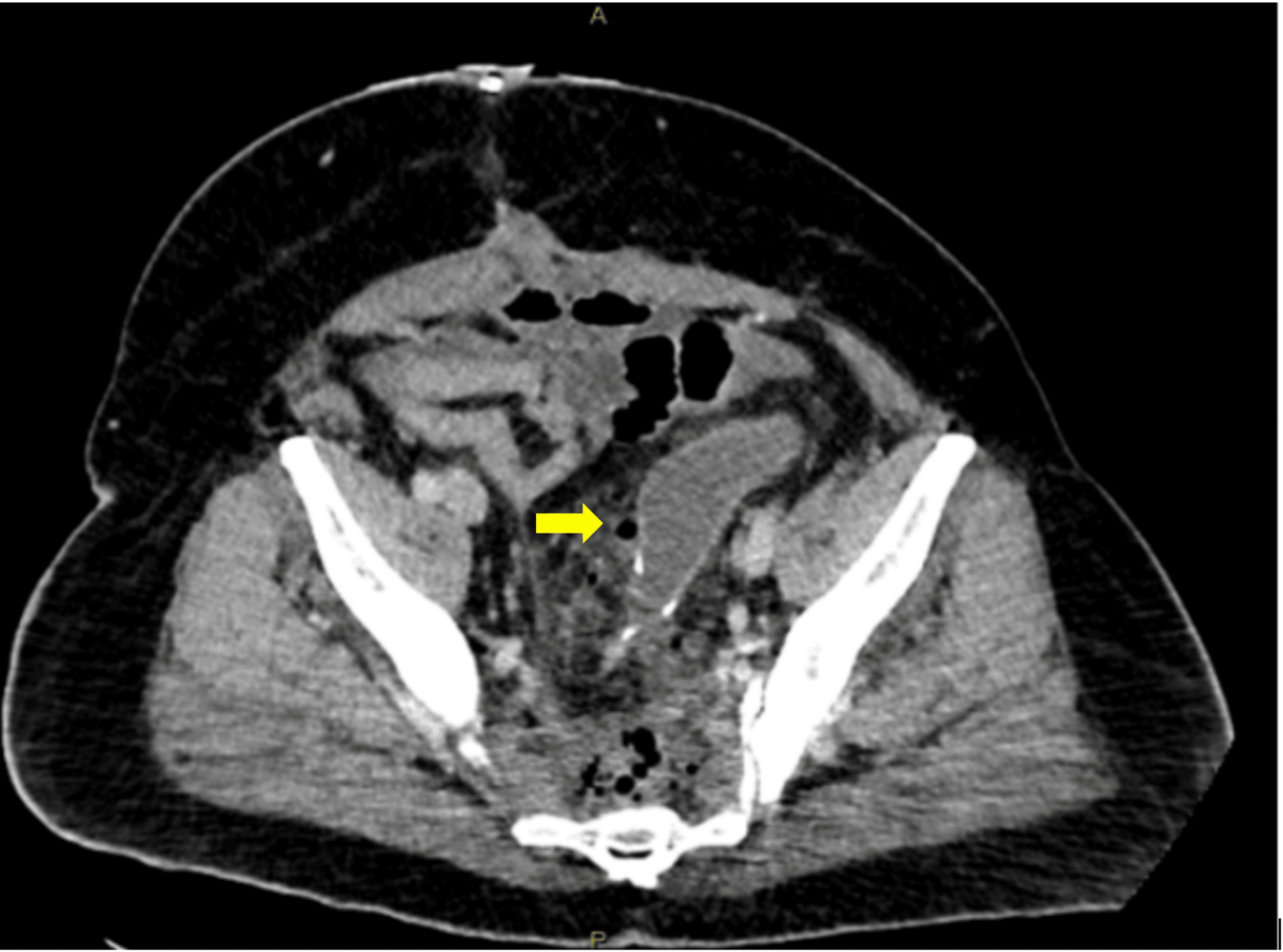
Axial CT abdomen pelvis showing gas locules adjacent to the anastomosis (yellow arrow)
